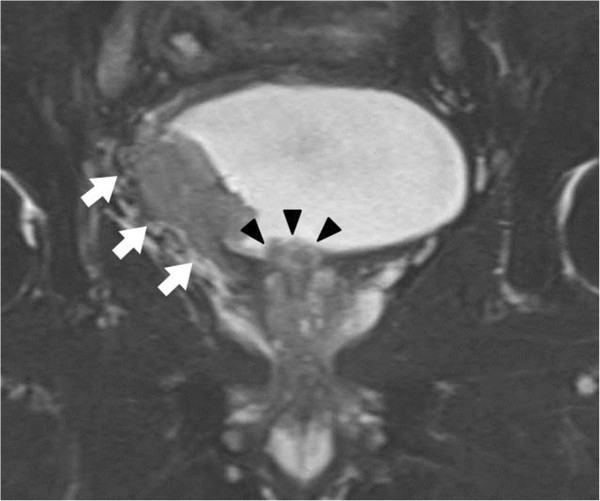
T2-weighted magnetic resonance imaging showed a locally-invasive tumor (arrows) on the right wall of the bladder and a prostatic urethral tumor that protruded into the bladder (arrowheads), without pelvic lymph node abnormalities.
